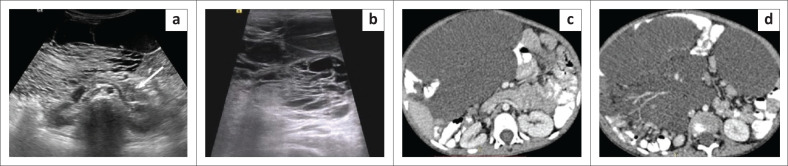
Mesenteric lymphangioma. Transverse grey scale ultrasound images (a, b) of the abdomen in a 3-year-old male child reveal an ill-defined multiloculated irregular-shaped thin-walled cystic lesion in the mesentery showing posterior acoustic enhancement. The lesion is located anterior to bilateral kidneys, pancreas (white arrow) and aorta. Multiple thin septations are noticed within the lesion creating a ‘cobweb’ appearance. Echogenic contents are seen within few loculi resulting in differential echogenicity of loculi. No calcification is noticed within the lesion. Axial contrast enhanced CT images (c, d) in the same patient reveal a multilobulated thin-walled fluid density cystic lesion in the mesentery anterior to pancreas and aorta. The lesion is insinuating between leaves of mesentery and bowel loops. Mesenteric vessels can be seen traversing through the lesion.
